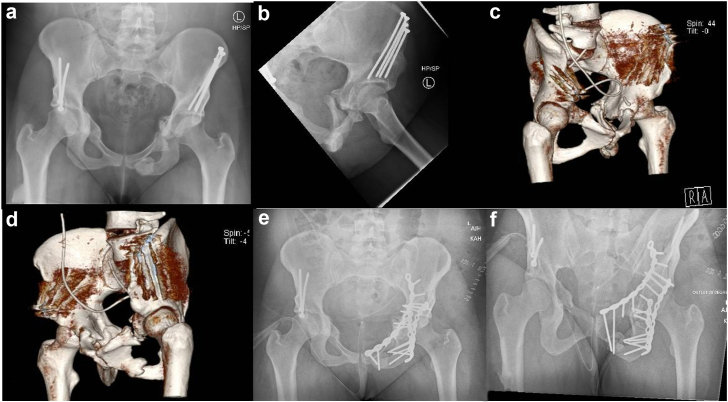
Imaging of a 28-year-old female. (a) Pelvis AP showing nonunion of pubic osteotomy and IP stress fracture, (b) Iliac oblique showing nonunion of PC stress fracture, (c) CT 3D reconstruction showing PC and pubic nonunion, (d) CT 3D reconstructions showing pubic and IP nonunion, (e) Pelvis AP showing postfixation construct performed through anterior intrapelvic approach and Kockher-Langenbeck, (f) Pelvis outlet view showing screws crossing IP stress fracture. 3D, 3-dimensional; AP, anterior-posterior.
